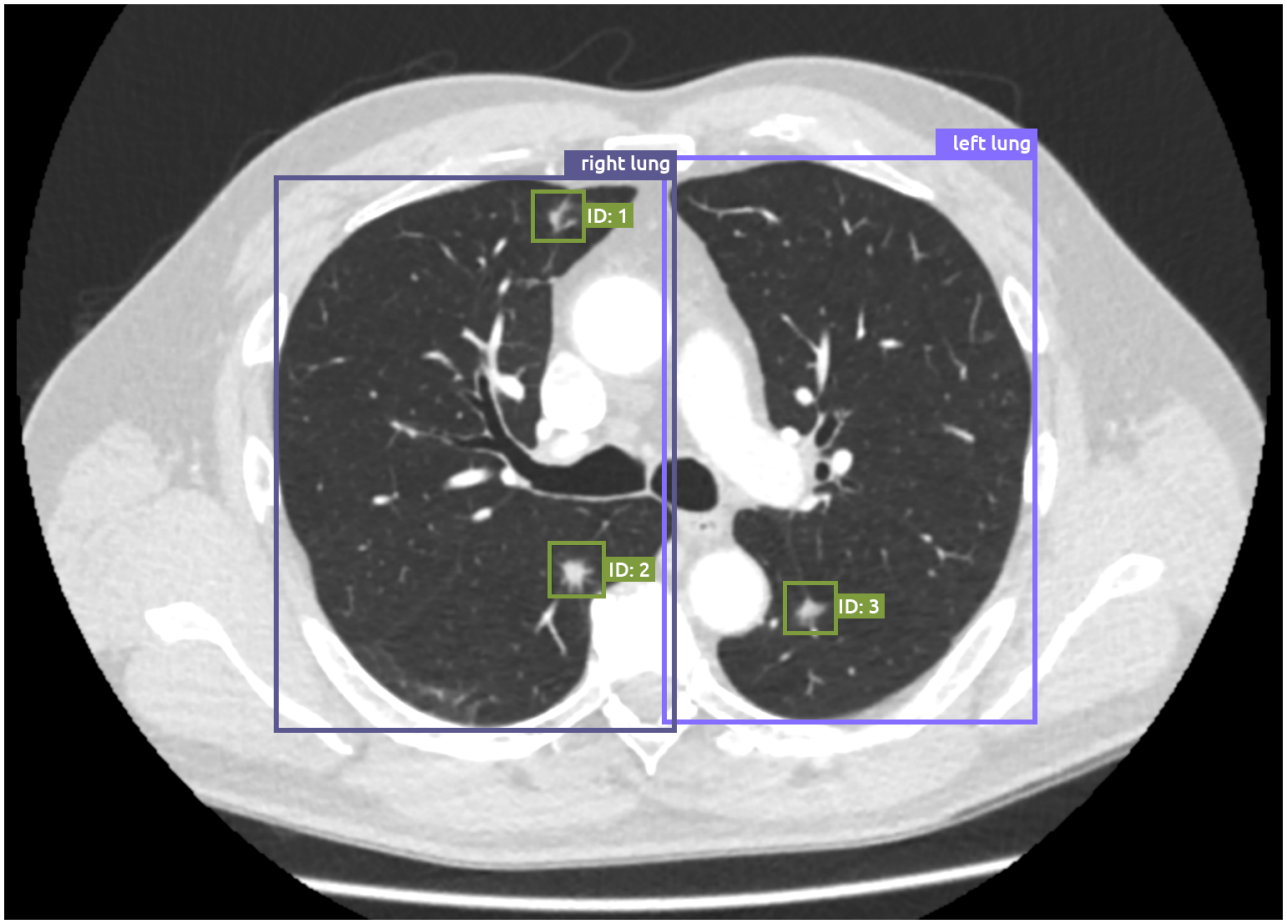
Example of the output of an artificial intelligence (AI) algorithm for lung localization and nodule detection on chest computed tomography (CT) imaging. The lung localization algorithm takes a slice of a CT scan as input, and produces a bounding box around the left lung and the right lung. The lung nodule detection algorithm takes a CT scan as input, and produces bounding boxes around detected lung nodules, which can be presented to radiologists as an aid for the detection of nodules in chest CT images
